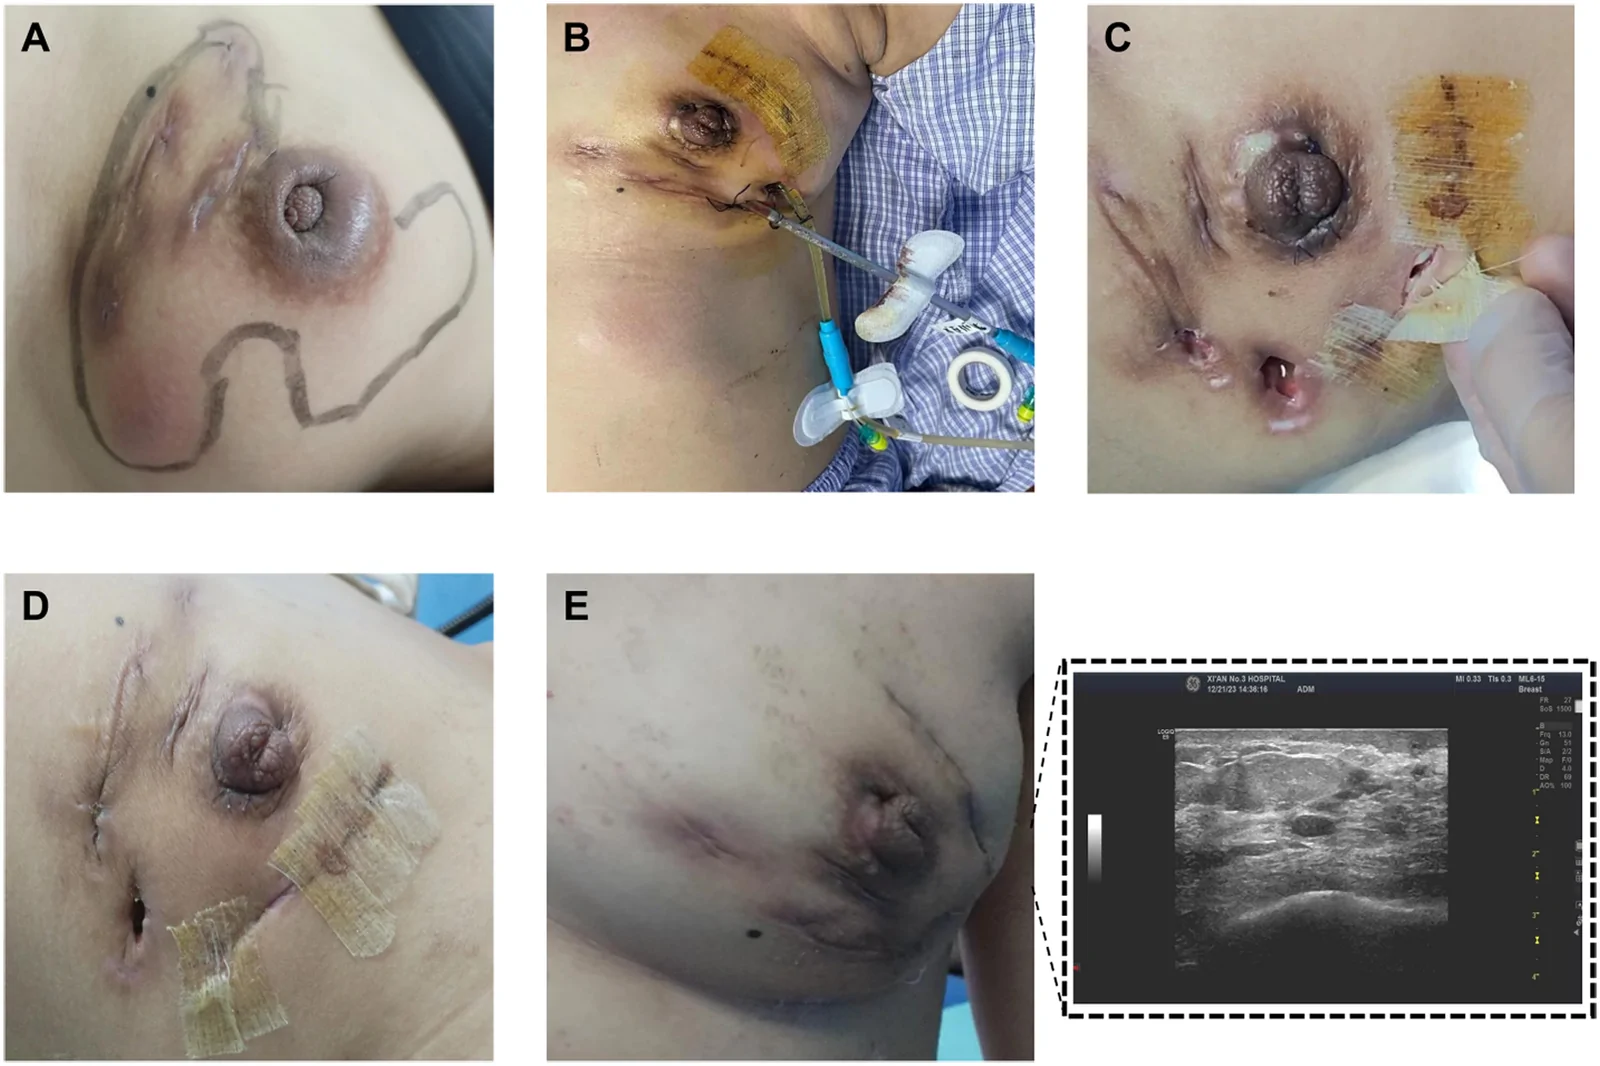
Clinical course and surgical management. (A) Preoperative appearance showing patchy erythema, ulceration, and sinus formation. (B) Early postoperative view with two irrigation drains in place. (C) Daily local irrigation with dexamethasone acetate through the drain using a tapering regimen. (D) Postoperative healing during the irrigation course. (E) Follow-up appearance with a satisfactory cosmetic result and no signs of recurrence, together with confirmatory ultrasonography showing resolution of the abscess (BI-RADS category 3).
